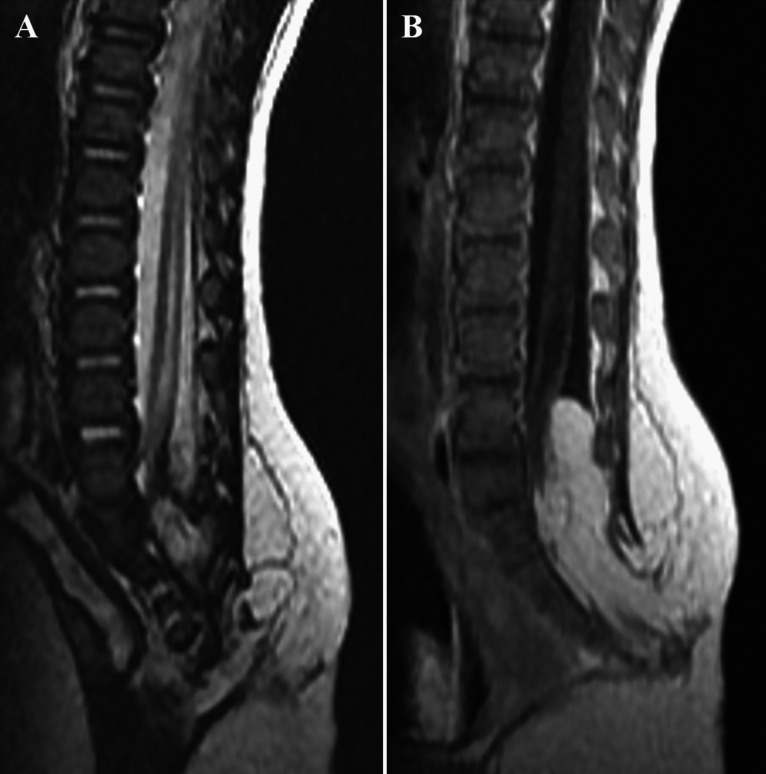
Magnetic resonance imaging (MRI) of patient 3. A T2-weighted sagittal MRI and B contrast-enhanced sagittal MRI. A, B It shows lumbosacral lipomatous malformation from L5 to S3 levels with diffuse syrinx
